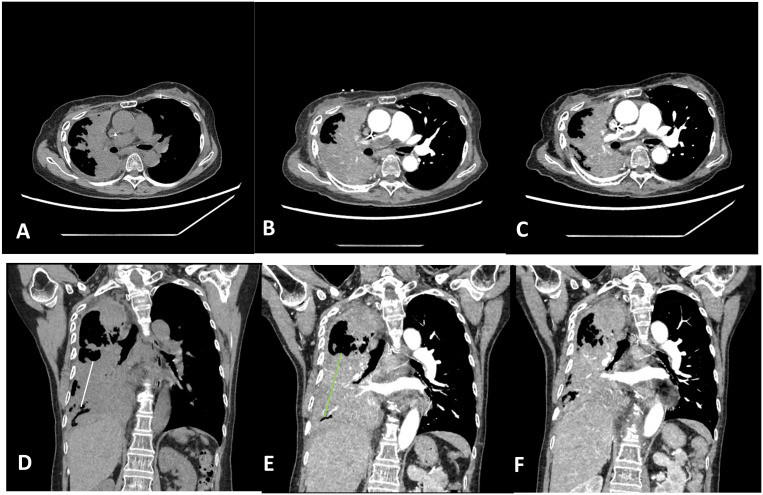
Chest computed tomography (CT) scans obtained at baseline and before and after fecal microbiota transplantation and the sixth cycle of etoposide and carboplatin chemotherapy. (A) Baseline chest CT shows near-total consolidation of the right lung with partially aerated areas in the right upper lobe. (B) Chest CT before fecal microbiota transplantation shows increased consolidation involving the right lower lobe. (C) Follow-up chest CT after fecal microbiota transplantation and the sixth cycle of chemotherapy shows newly developed aeration in the right lower lobe. (D–F) The linear extent of consolidation was measured on the same coronal slice and is indicated by white, green, and yellow lines ((D): 68.6 mm; (E): 91.4 mm; and (F): 76.7 mm, respectively).
